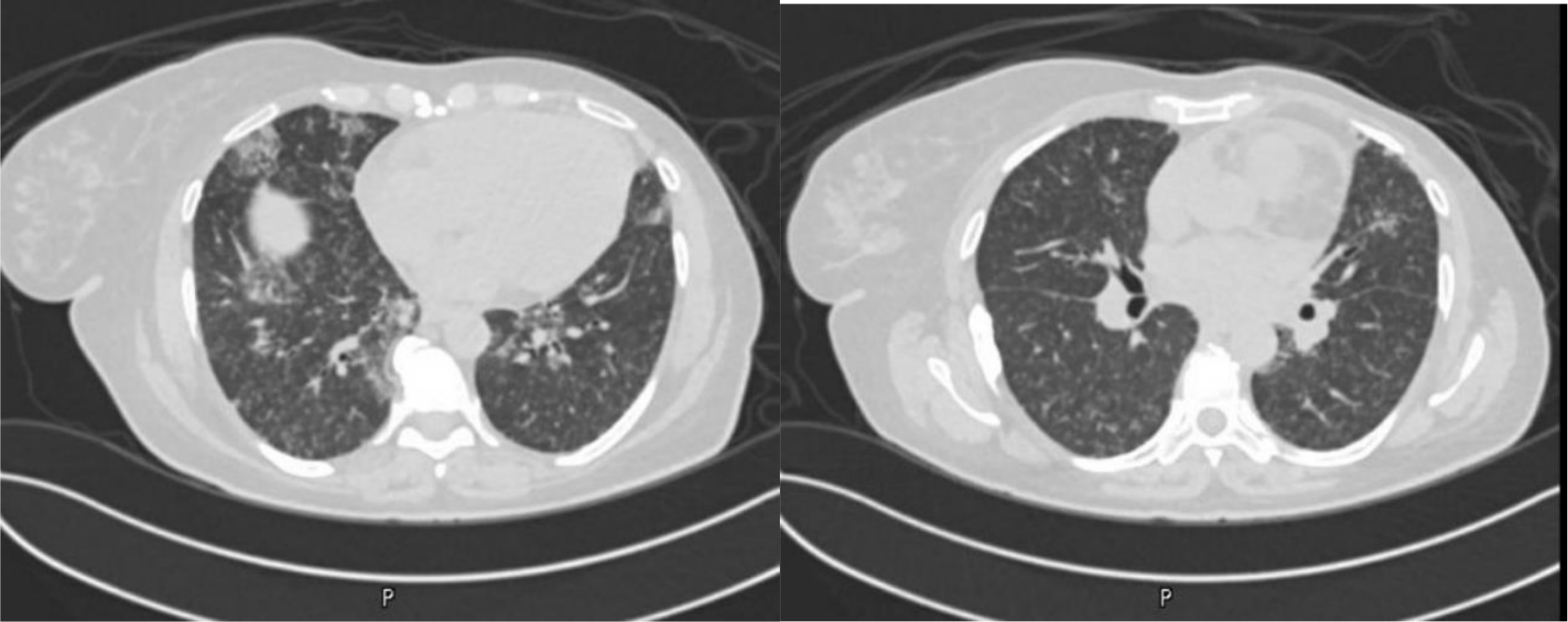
Chest CT scan showing diffuse numerous tiny nodules in both lung fields with areas of patchy GGOs and intralobular thickening more pronounced in both lower lobes.
